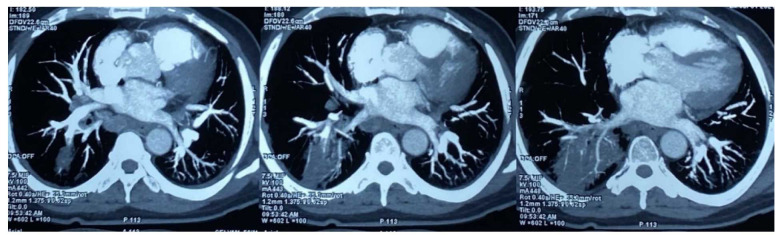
A 62-year-old male admitted for COVID-19-associated mucormycosis with sudden worsening of respiratory symptoms. Computed tomography pulmonary angiogram axial section shows central intraluminal thrombosis with complete occlusion of the posterior descending branch of the right pulmonary artery with consolidation changes in the posterobasal segment of the right lower lobe.
